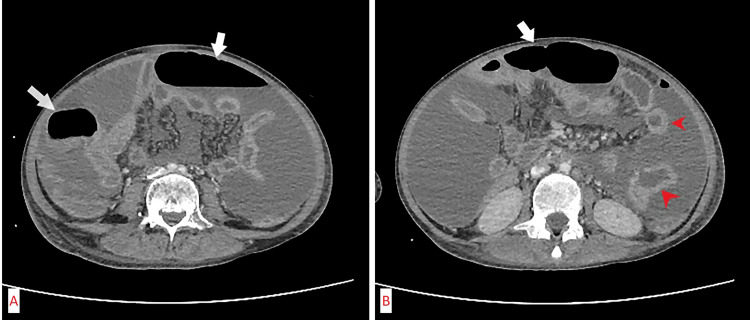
(A) Axial post-contrast CT scan of the abdomen showing signs of pneumoperitoneum (white arrows) and (B) axial CT scan of the abdomen showing postcontrast enhancement of small bowel wall as a sign of inflammation (pointed red arrows), free air in the abdomen (white arrow) after bowel perforation.
